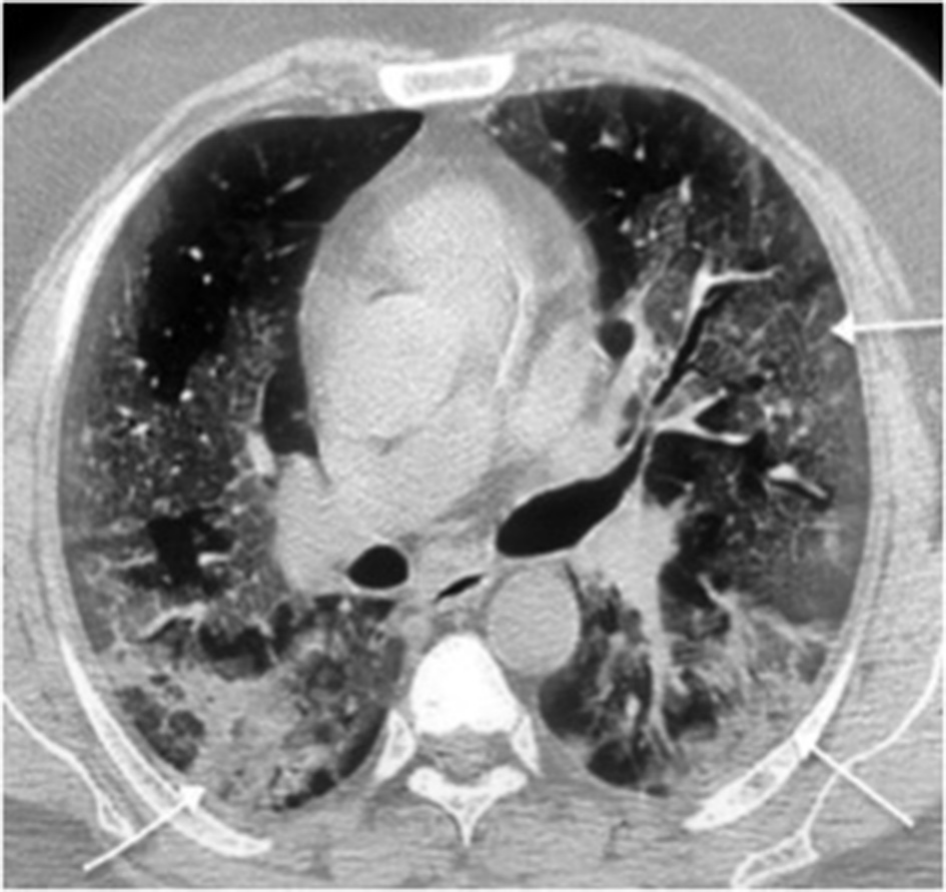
Chest CT, lung window axial section shows coalescing ground glass opacities pointed by the horizontal arrow. The slanting arrows point to bilateral areas of predominant consolidation.
